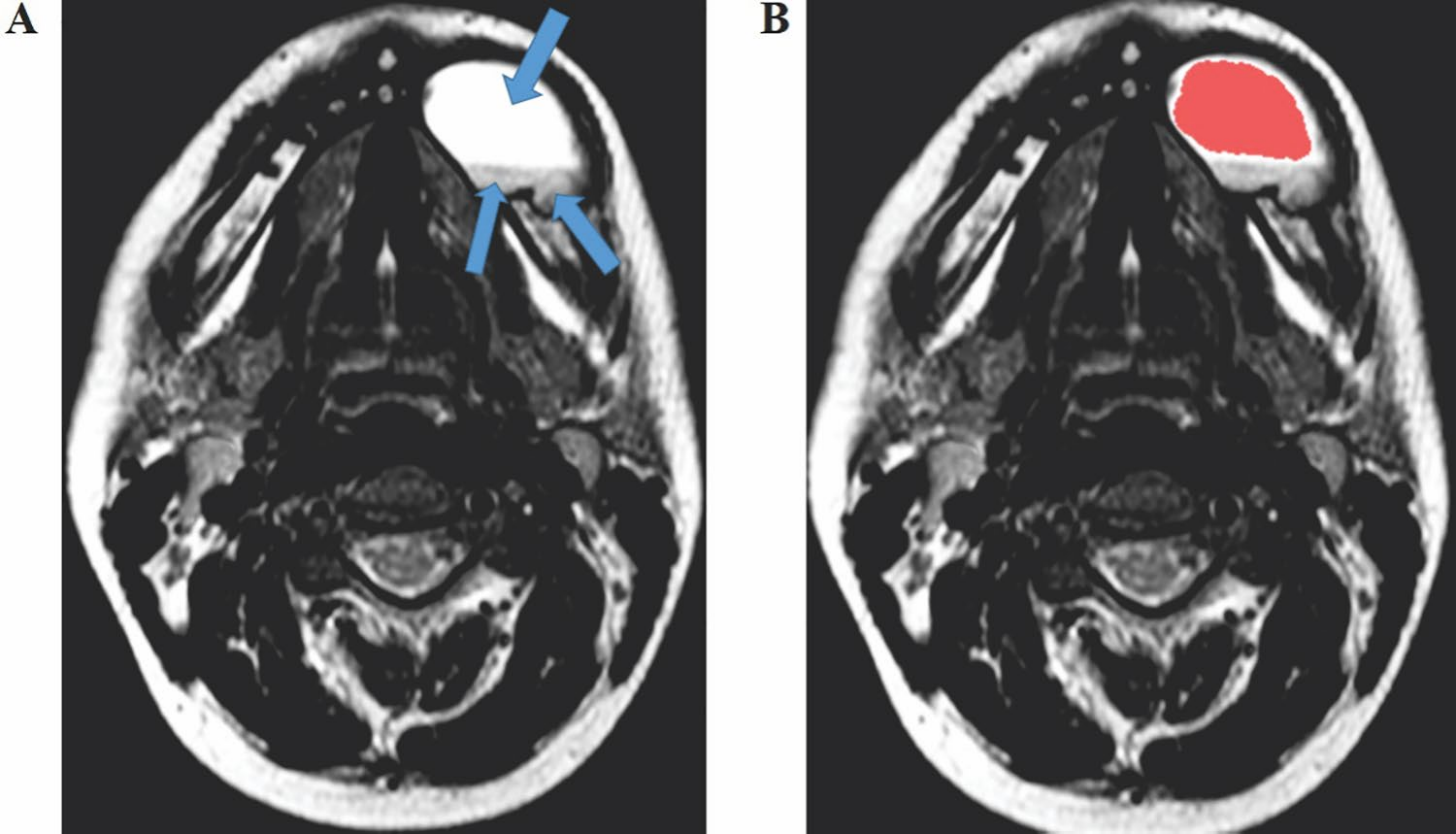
(A) Ameloblastoma located in the mandibular body on the left side. Arrows represent regions with variable signal intensity, which are characterized by hypersignal representing higher aqueous content, a characteristic of T2-weighted sequences. (B) Segmentation process represented by the red contour. Only the most homogeneous portion of the lesion was segmented, that is, the region shown by the hypersignal.
